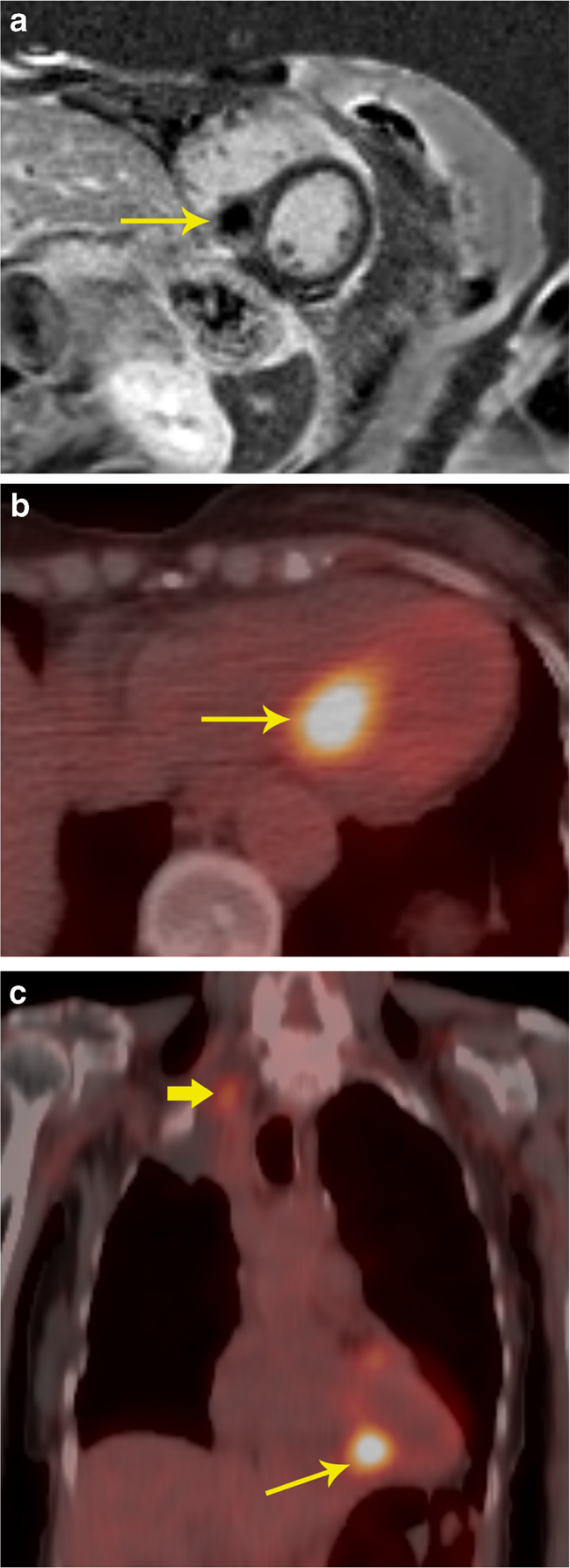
Staging. a Short-axis delayed enhancement MRI in a 72-year-old patient shows a mass in the ventricular septum (arrow) that has peripheral enhancement and central non-enhancement. b FDG-PET/CT image shows intense uptake in the mass in the ventricular septum, indicating that this is malignant. c Coronal FDG PET/CT image in the same patient shows the septal lesion (thin arrow). In addition, there was also a hypermetabolic right apical lung mass (thick arrow), consistent with lung cancer. The septal lesion is a metastasis. There were also metastatic lesions in the spine
